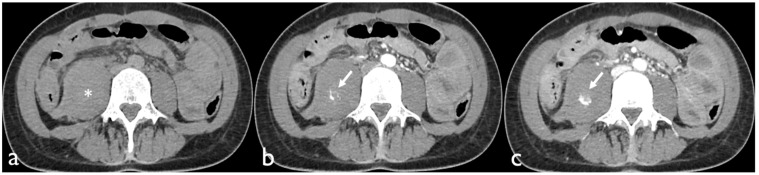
Non-contrast CT image (a) of a patient presenting with a hematoma of the right iliopsoas muscle extending into the retroperitoneal space (*). Contrast-enhanced axial CT images show active bleeding in the arterial (b) and portal phase (c).
